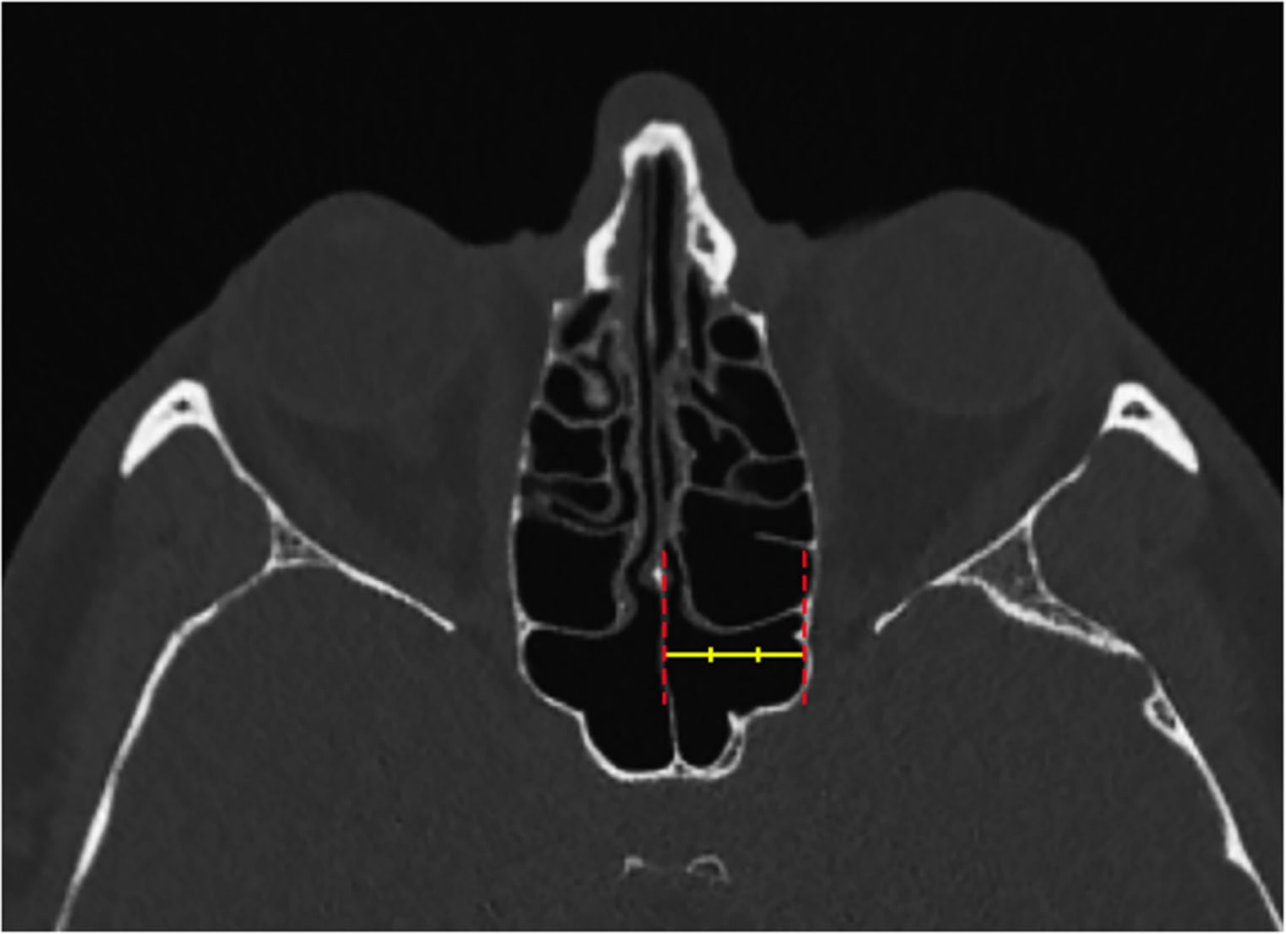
Axial CT image demonstrating the horizontal reference line divided into three equal segments to identify the horizontal position of the sphenoid sinus ostium. The yellow line represents the horizontal reference line from the medial margin of the ostium to the nasal septum. Red dotted lines indicate the midline of the nasal septum and the lateral boundary of the sphenoid sinus
